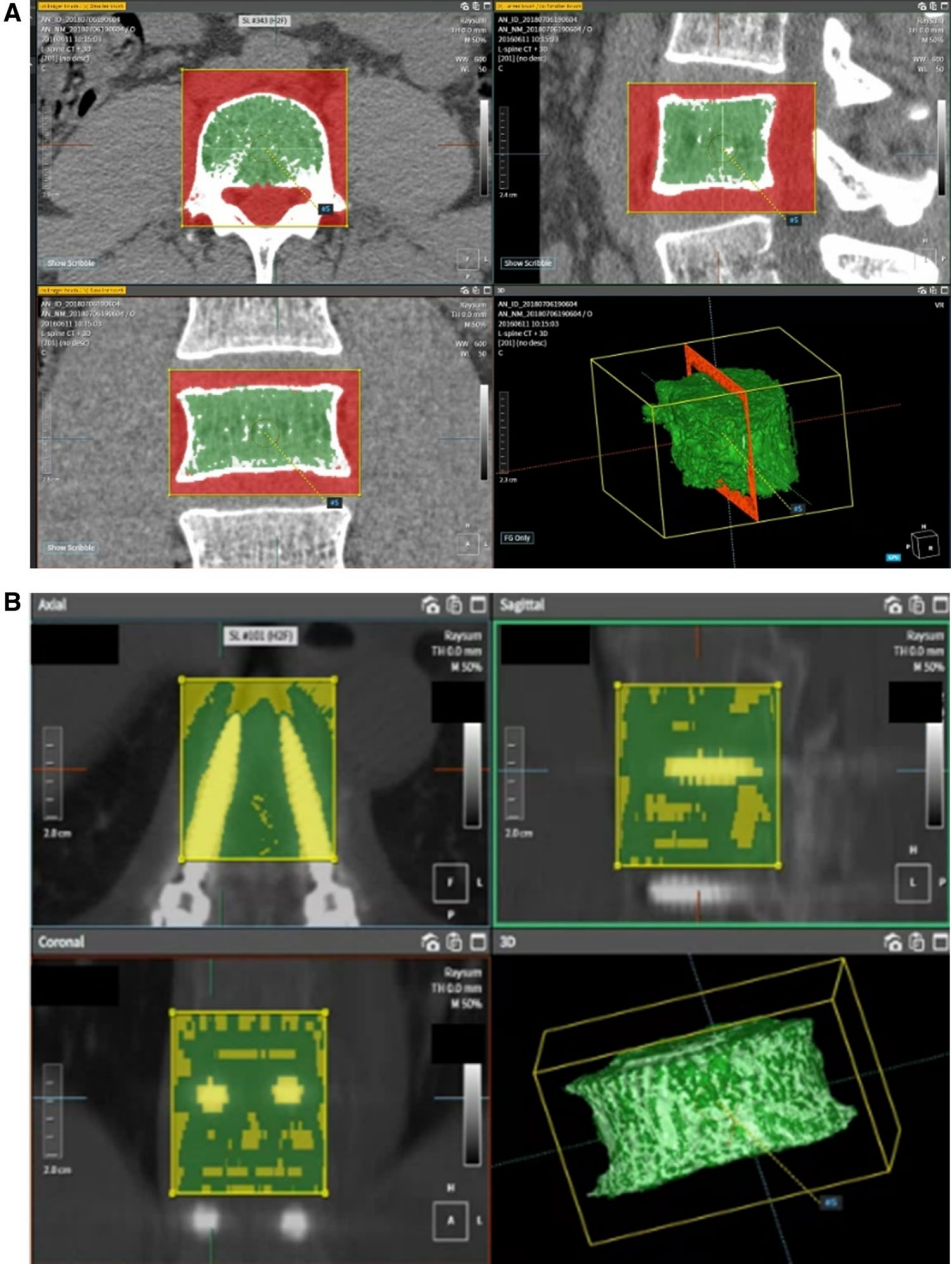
(a) Hounsfield Units (HU) measurement of vertebral body without pedicle screw from computed tomography using the AVIEW software. An upper limit of 1500 HU was used to exclude metal artifacts caused by screws and cortical bone, and a lower limit of 100 HU was used to exclude disc and other soft tissues. (b) Vertebral body with pedicle screw HU measurement. Upper and lower limit of HU get rid of pedicle screw and metal artifacts.
